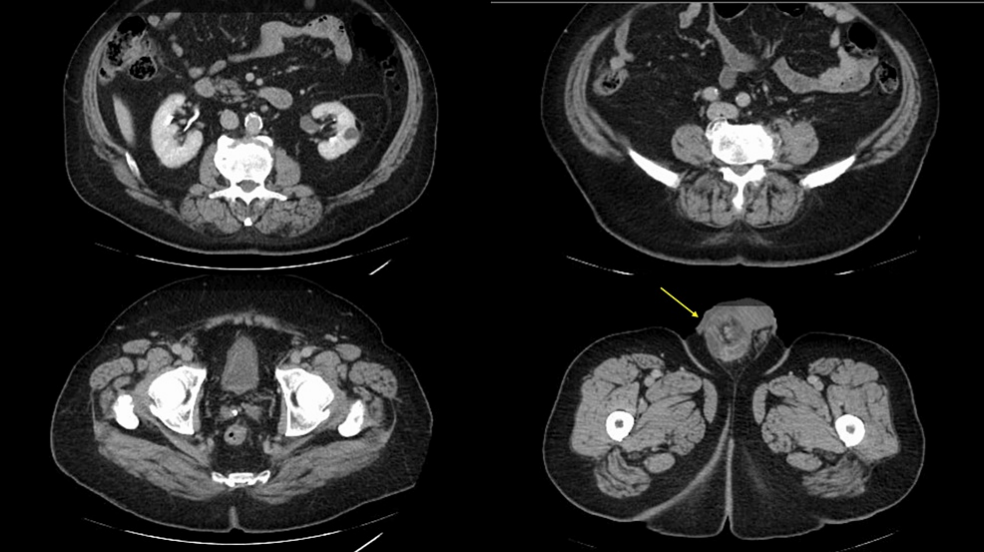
CT of the abdomen and pelvis showing right inguinal hernia without metastatic disease (arrow) CT: computed tomography
